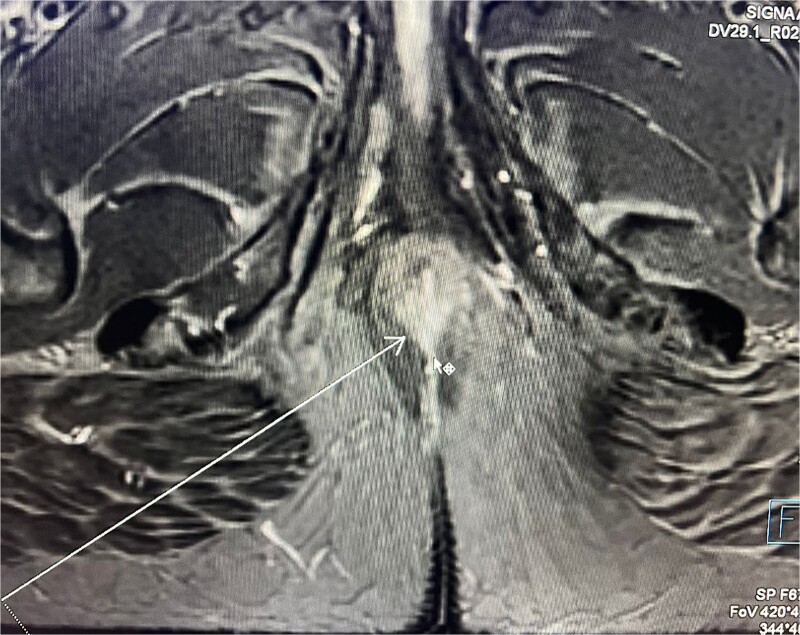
Abdominal and pelvic magnetic resonance imaging. The white arrow points to a 16 x 22 mm mass in the perianal region, where asymmetric thickening affecting both the external and internal sphincters is evident, located just at the anodermic junction and extending into the levator ani muscles.
